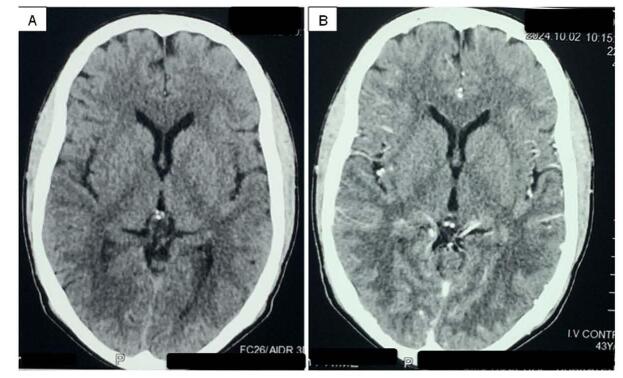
(A) Non-contrast CT and (B) contrast enhanced CT brain at six months follow up showing no tuberculoma.
